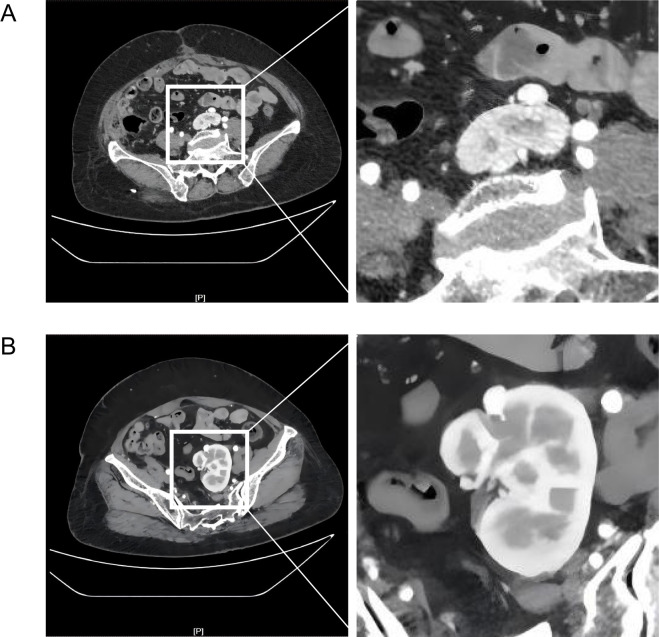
Preoperative contrast-enhanced CT localization of the pelvic ectopic kidney. (A) The upper pole of the left pelvic ectopic kidney is situated at the bifurcation of the left internal and external iliac arteries. (B) The overall anatomical position of the left pelvic ectopic kidney is at the level of the sacral promontory.
